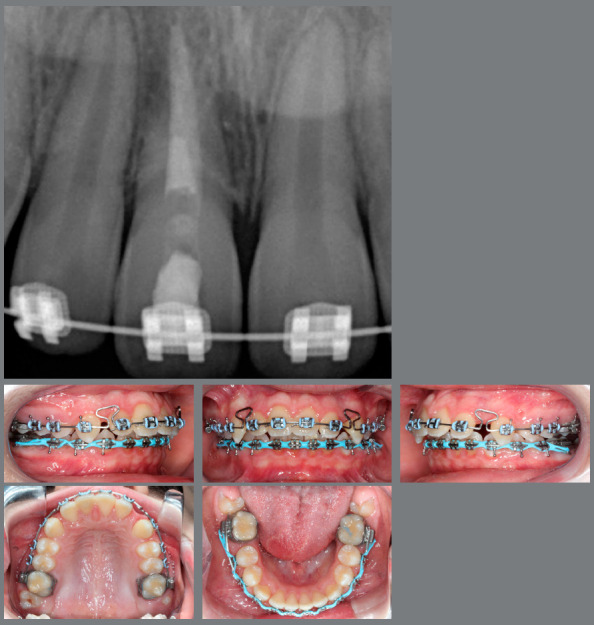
Radiograph and intraoral photographs showing the ankylosed tooth and the loss of anchorage during space closure.
